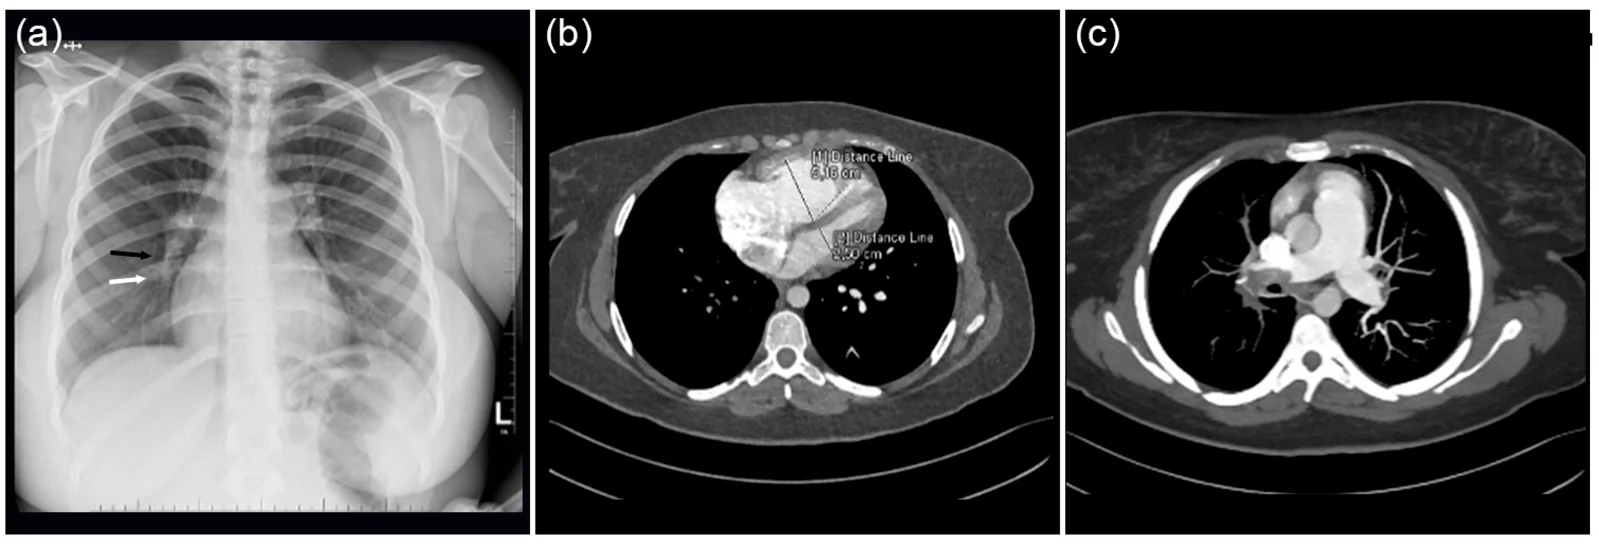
Chest radiograph and CT pulmonary angiography. (a) Posteroanterior chest radiograph showing an enlarged right descending (interlobar) pulmonary artery (black arrow; Fleischner’s sign) with an abrupt reduction in calibre distally (white arrow; Chang’s sign), both suggestive of pulmonary embolism. (b) Axial contrast-enhanced CT image demonstrating right ventricular enlargement, indicating right ventricular strain. (c) Axial contrast-enhanced CT image at the level of the pulmonary artery bifurcation, cranial to (b), showing filling defects in the pulmonary arteries, more extensive on the right.
